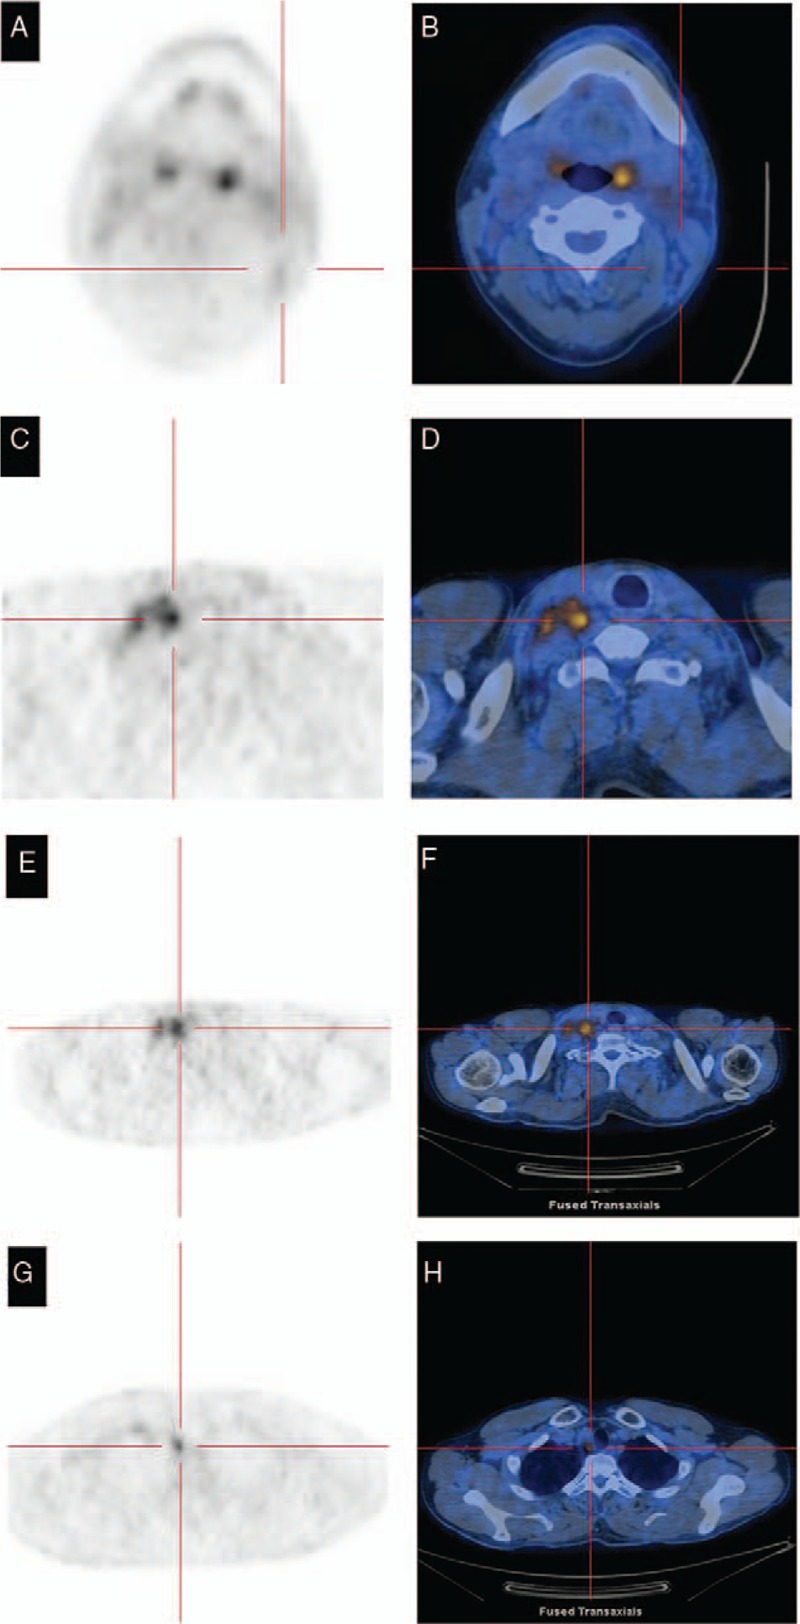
^[18]^Fluorodeoxyglucose positron emission tomography–computed tomography scan of the present lymphocyte-rich classical HL: fluorodeoxyglucose avidity were normal or slight increase in left tonsil and left cervical lymph nodes (A and B), because this scan was carried out after the resection of left tonsil and cervical lymph nodes. The standard uptake value (SUV) of fluorodeoxyglucose of multiple lymph nodes of the upper right clavicle (C and D), under right clavicle (E and F) and mediastinum (G and H) were high, with the maximum value 7.5. HL = Hodgkin lymphoma.
